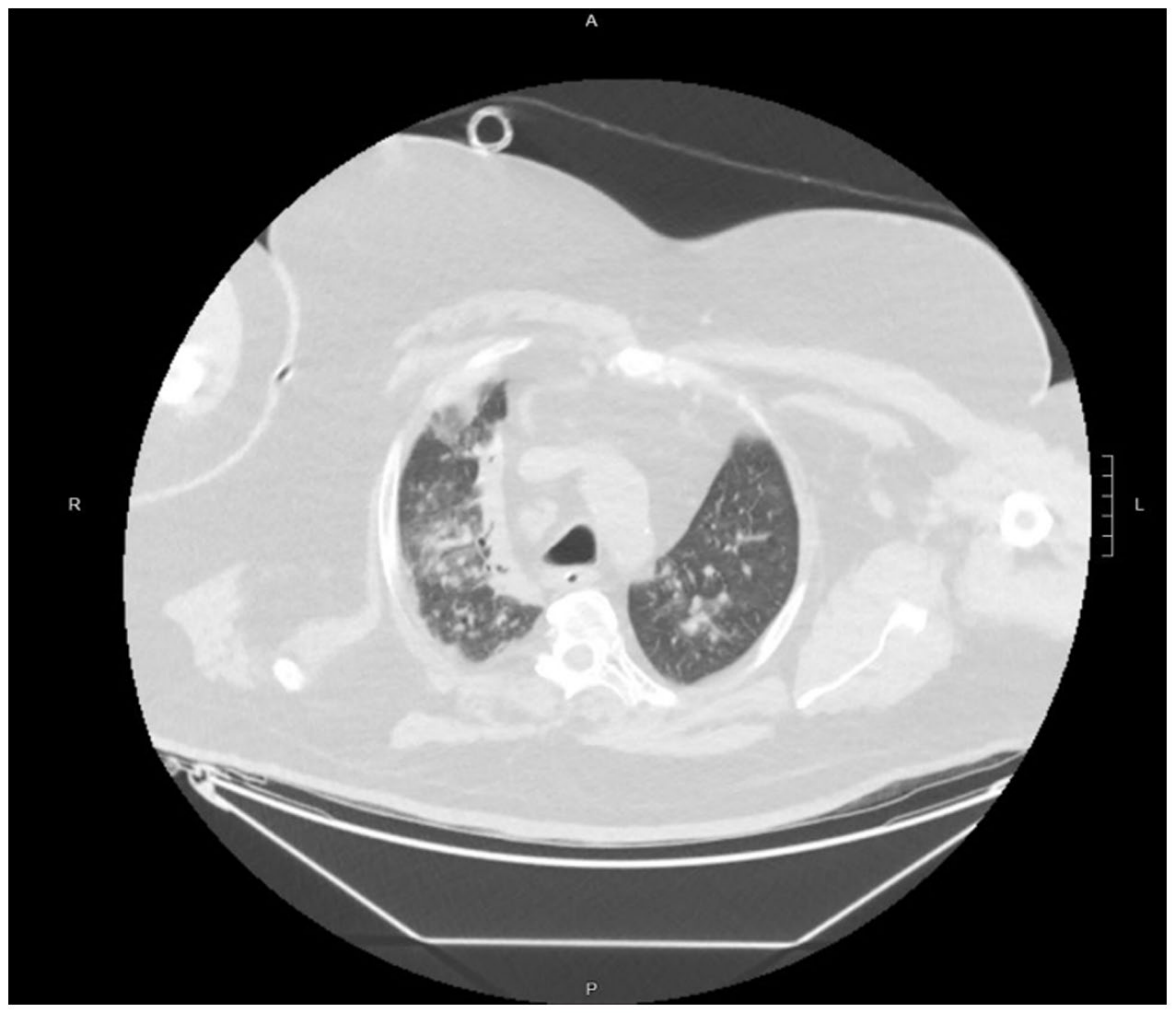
Chest computed tomography (CT) on ICU admission. Extensive confluent parenchymal consolidations with air bronchograms are visible in the right lung, associated with diffuse ground-glass opacities and “tree-in-bud” patterns in the left upper lobe—findings consistent with bilateral viral pneumonia.
